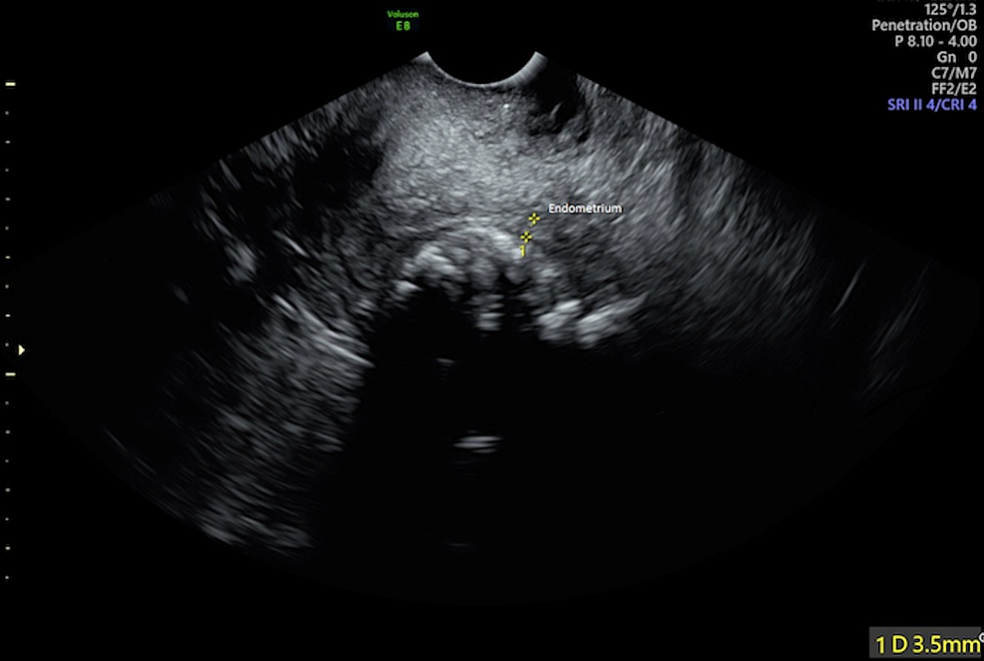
Ultrasound image displaying an endometrial thickness of 3.5 mm with normal adnexa.
